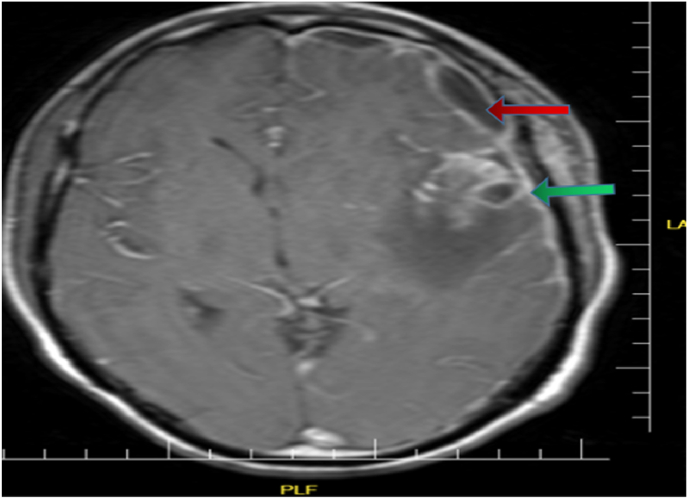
A contrast-enhanced T1 axial MRI showed a cranial epidural abscess. on the left side of the epidural space.
